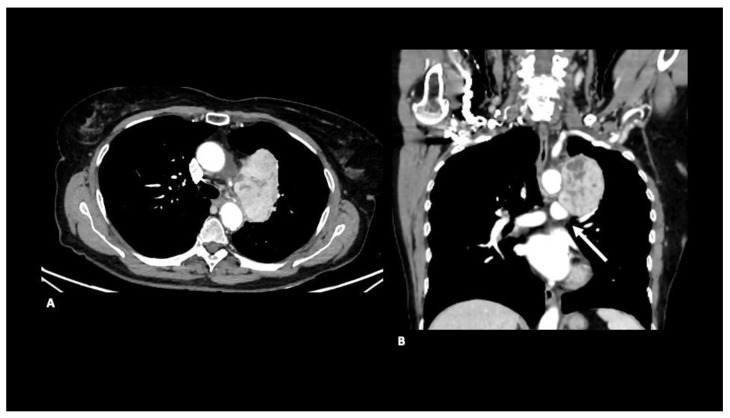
Axial (A) and coronal (B) contrast-enhanced CT images show a large, heterogeneous mediastinal mass that extends into the aortopulmonary window and compresses the left pulmonary artery. Note the large size and the heterogeneous attenuation, standard features of leiomyosarcoma.
